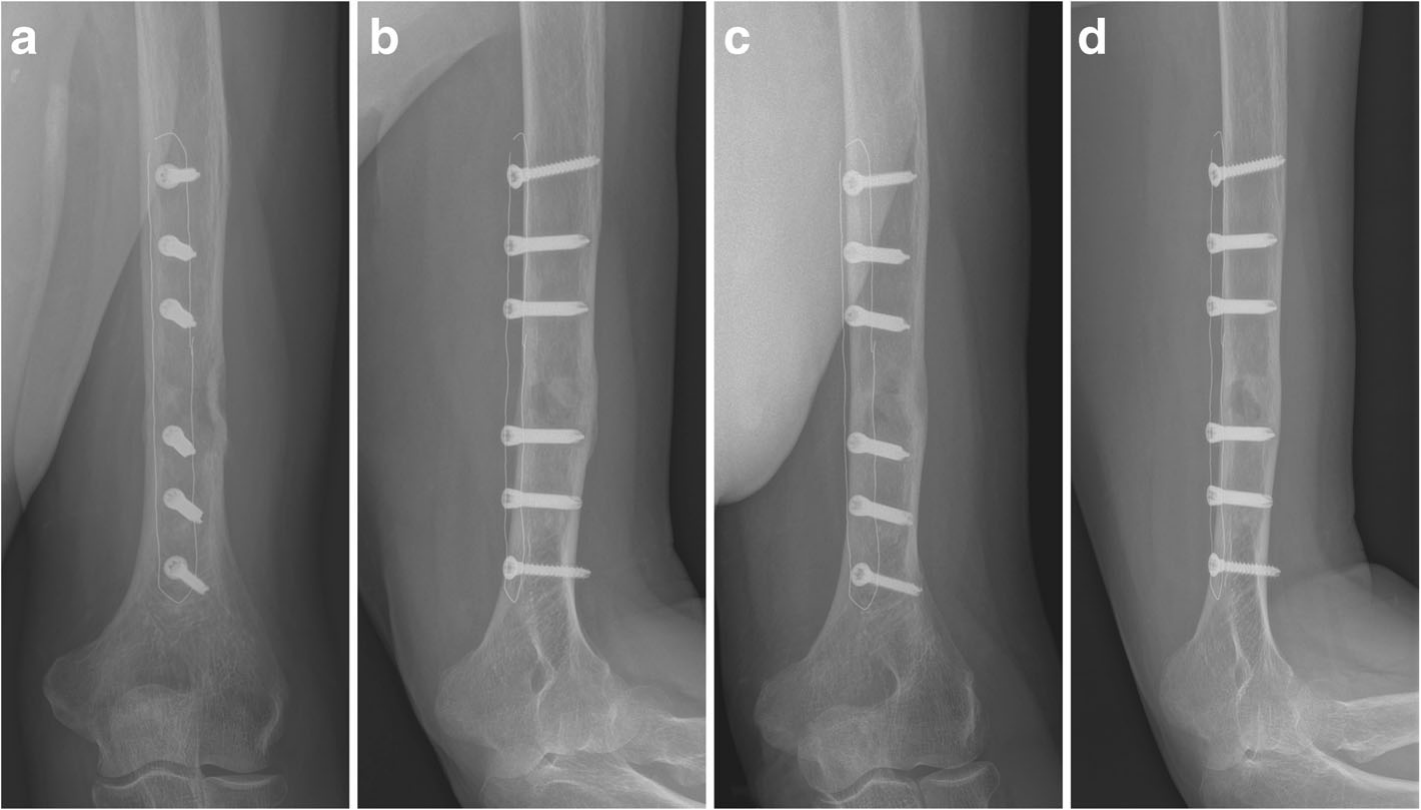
The postoperative x-ray at 6 weeks (a, b) and 6 months of follow-up (c, d) shows bridge plating of the lesion and anatomic bone alignment and eventually completed osseous consolidation
